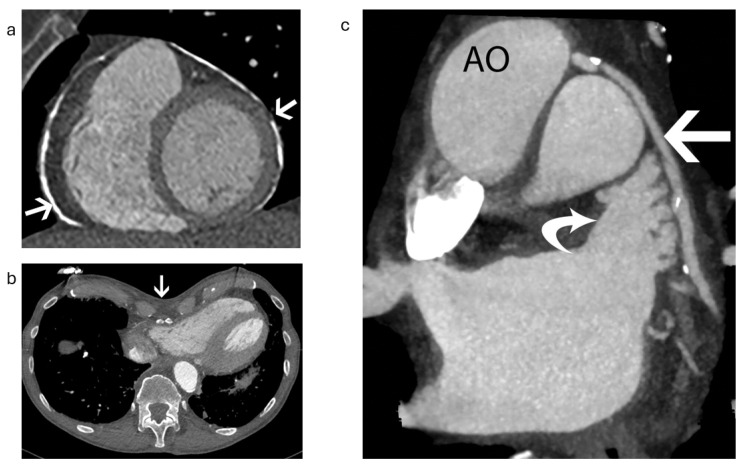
Contraindications for placement of hybrid endocardial–epicardial devices in three patients. AO stands for aorta (a) Short-axis reconstructed CT image in a 47-year-old man shows circumferential pericardial calcification (arrows). (b) Axial CT image in a 43-year-old man shows a severe pectus excavatum deformity (arrow) with a Haller index score of 4.5. (c) Coronal reconstructed CT image in a 72-year-old man shows a venous bypass graft from the aorta to the obtuse marginal artery (straight arrow), which is situated directly adjacent to the LAA (curved arrow). CT: Computed tomography, LAA: Left atrial appendage. Adapted with permission from Rajjah et al. [16].
